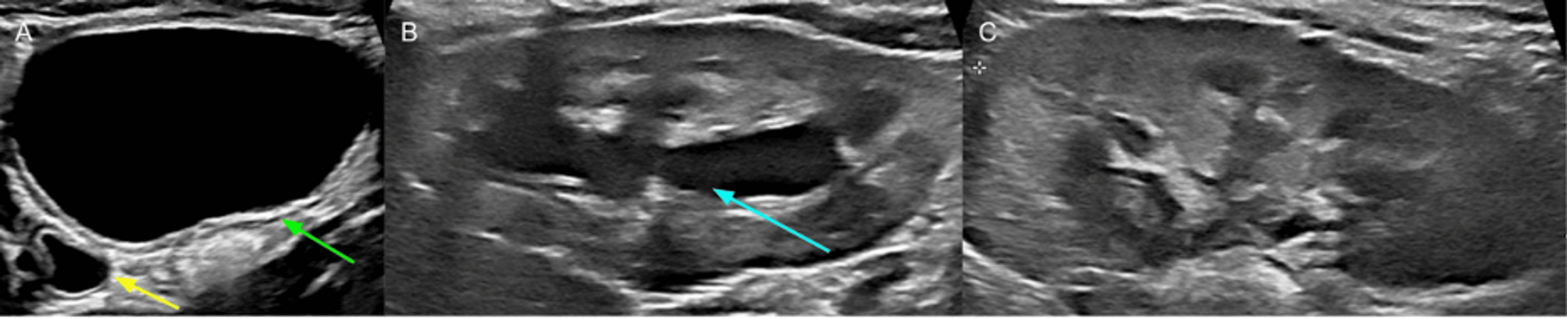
Renal and bladder ultrasound one month postoperatively (A) Transverse view demonstrating a full bladder (green arrow) and distal right ureteral dilation (yellow arrow). (B) Sagittal view of the right kidney demonstrating improved hydronephrosis (blue arrow). (C) Sagittal view of the left kidney demonstrating resolution of the hydronephrosis.
